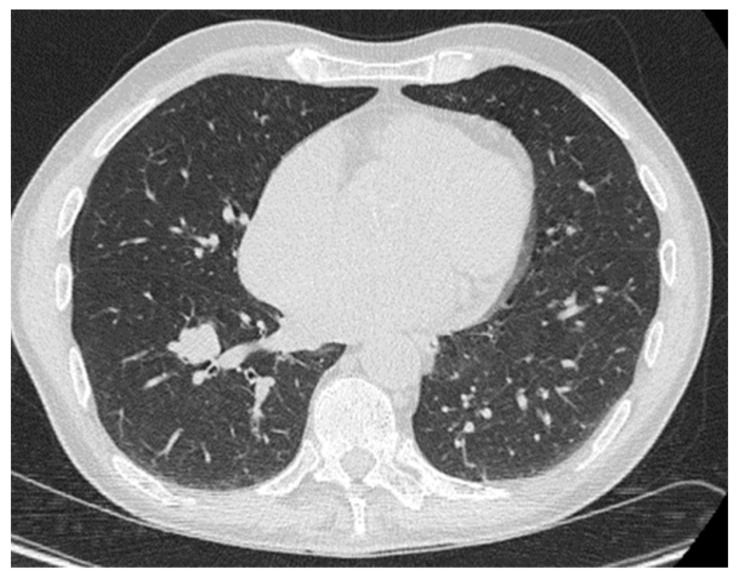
Biopsy-proven lung EP, case from our institution. Axial CT image demonstrates a lobulated lung nodule in the right lower lobe.
